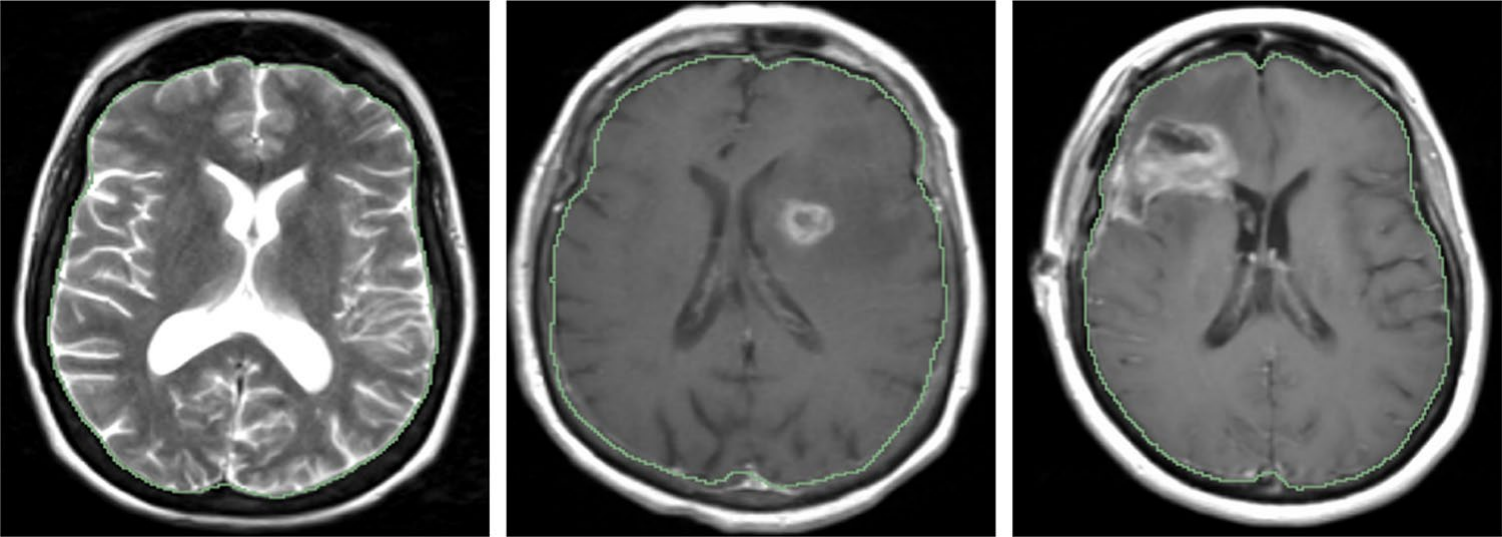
Showcases of skull stripping in different stage: healthy brain T2-weighted MRI (left), pre-operative T1-ce brain tumor MRI (middle), and post-operative T1-ce brain tumor MRI (right). The green contour is the boundary of skull stripping using the proposed method.
